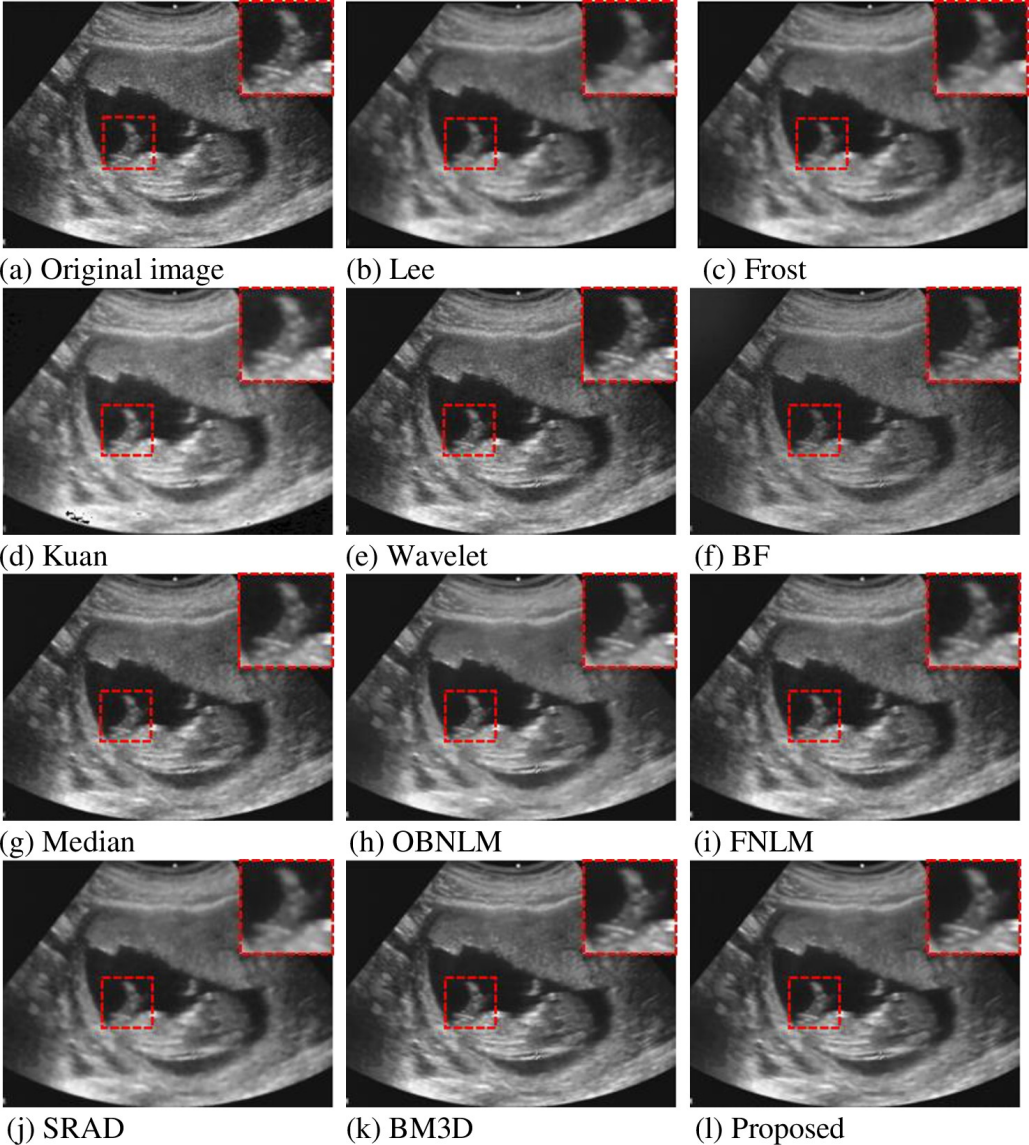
Effect of fetal ultrasound denoising.
